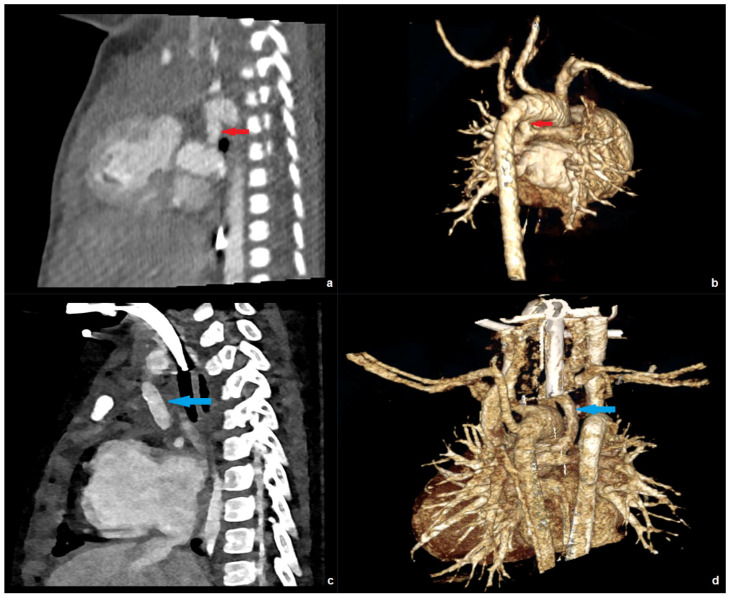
EID-CT images of a patient with hypoplastic left ventricle and pulmonary atresia at the age of 10 days: sagittal (a) and 3D right posterior oblique (b) reconstruction depicting the patent ductus arteriosus (red arrow). PC-CT images of the same patient at the age of 17 month sharply depicting a Blalock-Taussig shunt (blue arrow) in the sagittal (c) and 3D right posterior oblique (d) view.
